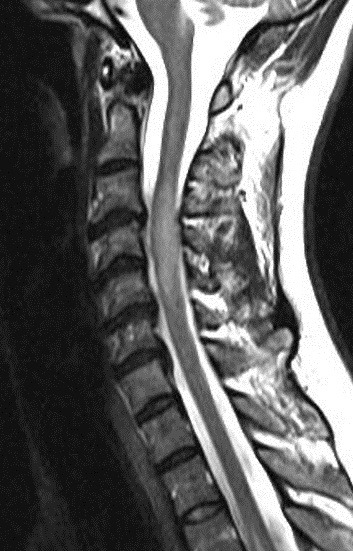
MRI spine showing hyperintensity in cervical cord C5 to C7 level (3)
